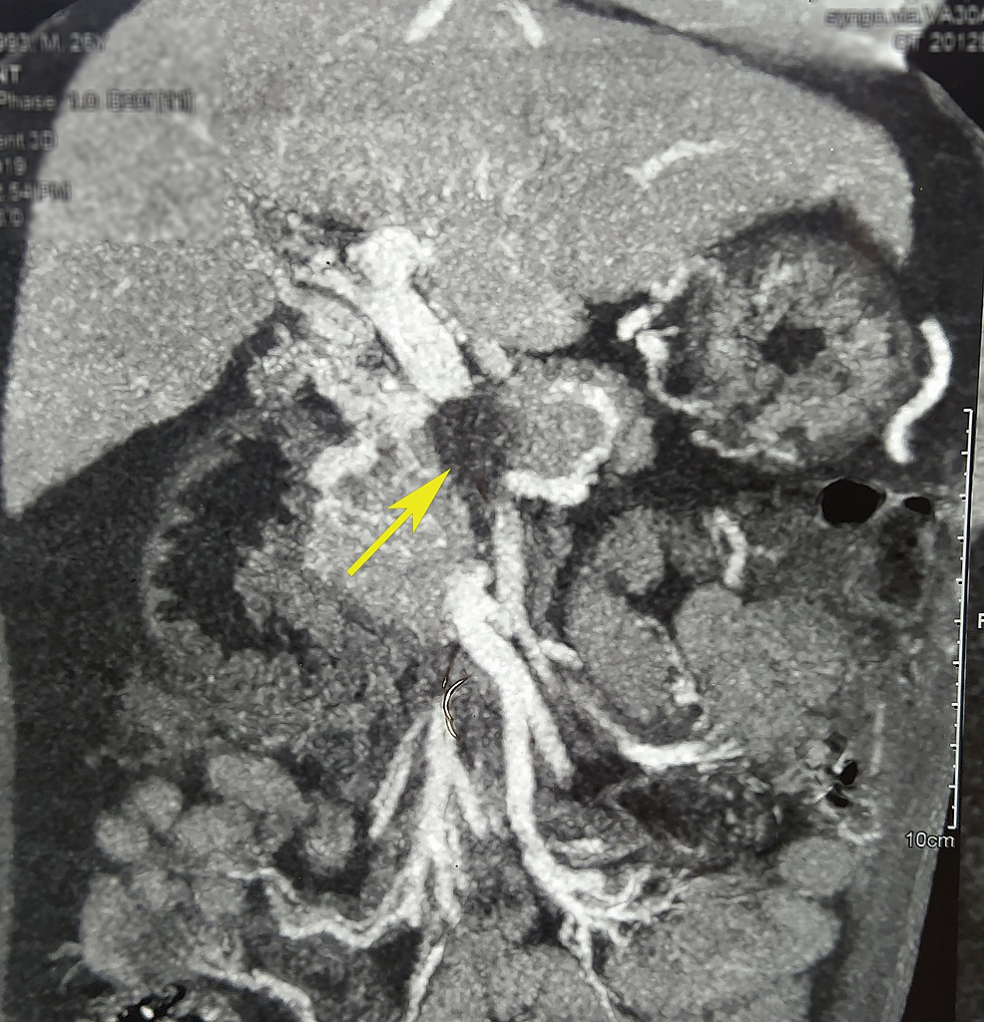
Coronal view of contrast CT (venous phase) showing thrombus in the main portal vein and the distal superior mesenteric vein (yellow arrow). CT, computed tomography
